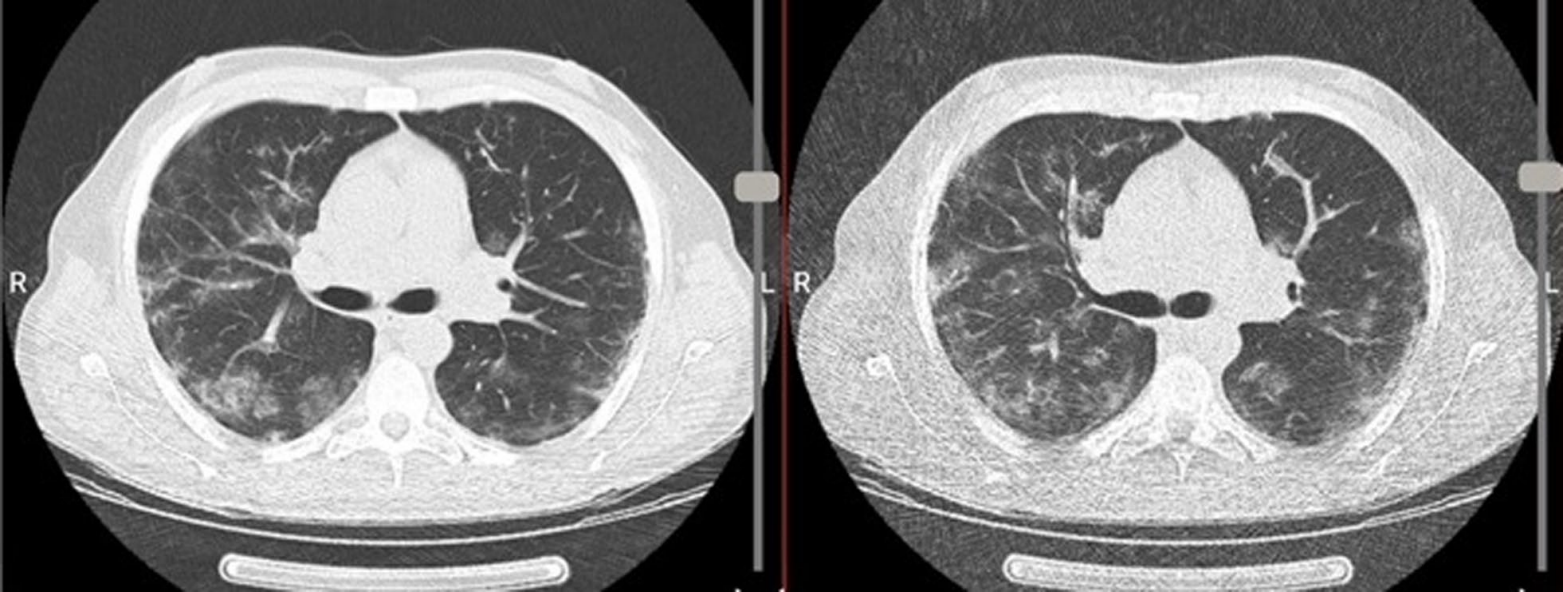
Bilateral multifocal subpleural and peribronchovascular ground-glass opacities in low-dose (left image) and ultra-low-dose (right image) CT scans typical for COVID-19 infection
